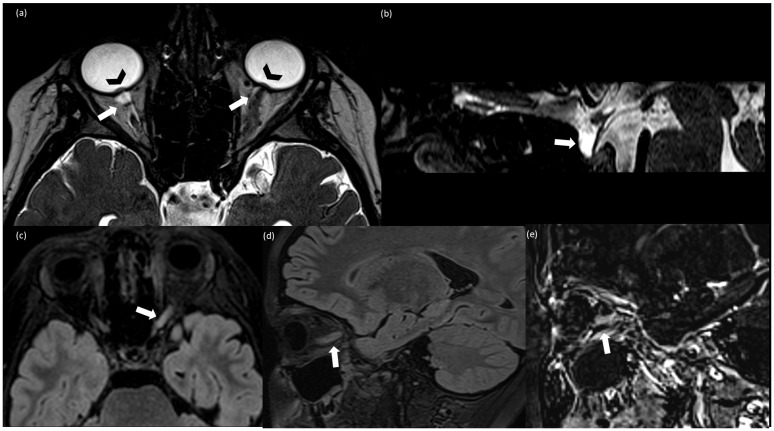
(a) Axial T2-weighted CISS (Constructive Interference Steady State); (b) sagittal T2-weighted CISS; (c) axial FLAIR (Fluid Attenuated Inversion Recovery; (d) sagittal T2 FLAIR; (e) sagittal subtraction images between post-contrast and pre-contrast T1 weighted MPRAGE. (a,b) Finding consistent with idiomatic intracranic hypertension (IIH): bilateral prominent subarachnoid space around the optic nerves (arrow), more evident on the right side because of the left optic nerve swelling, intraocular protrusion of the optic nerves (arrowhead) (a), and partially empty sella (arrow) (b); (c–e) findings consistent with optic neuritis (ON): T2-FLAIR hyperintensity and swelling of the left optic nerve (c,d) associated with contrast enhancement (e).
